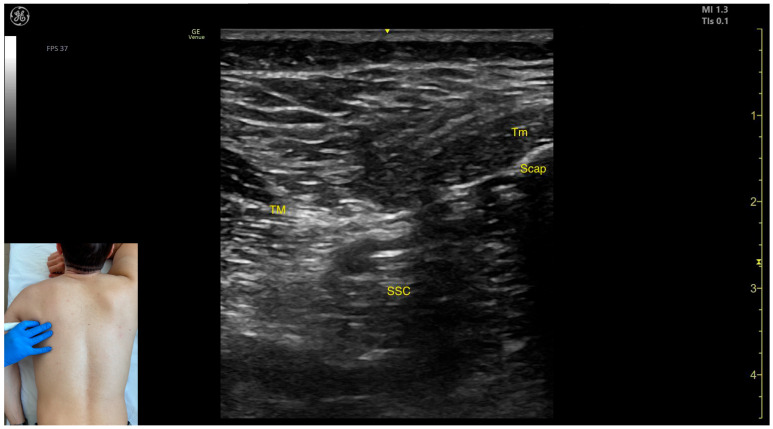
Ultrasound anatomy of the teres major (TM) with key landmarks: TM—teres major; Tm—teres minor; SSC—subscapularis; and Scap—scapula.
